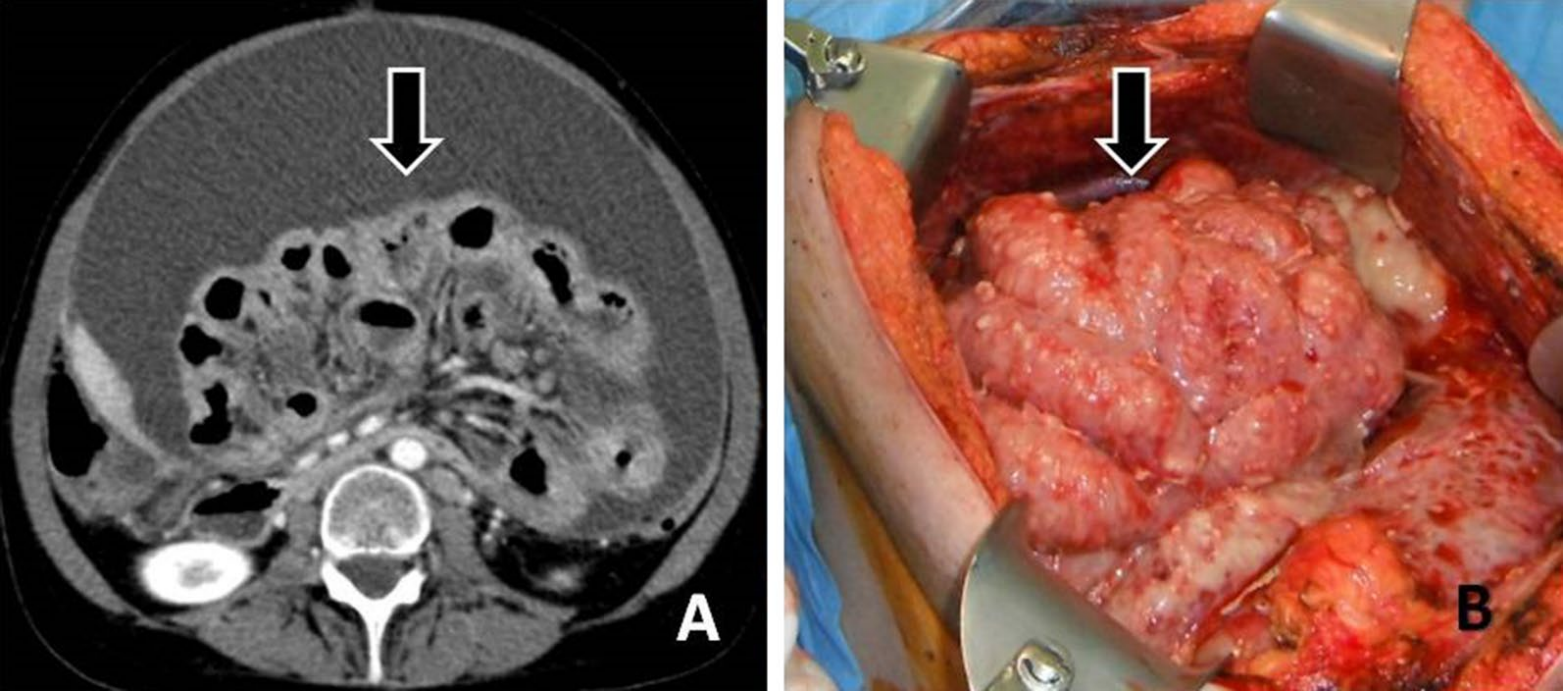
a Contrast enhanced CT image demonstrates shrinkage and distortion of the mesentery—“frozen mesentery”—(arrow) along with irregular thickening of the wall of intestinal loops (“layered-type” involvement of small bowel). b The extensively involved mesentery and intestinal loops are seen as a "cauliflower" mass at surgery (arrow)
